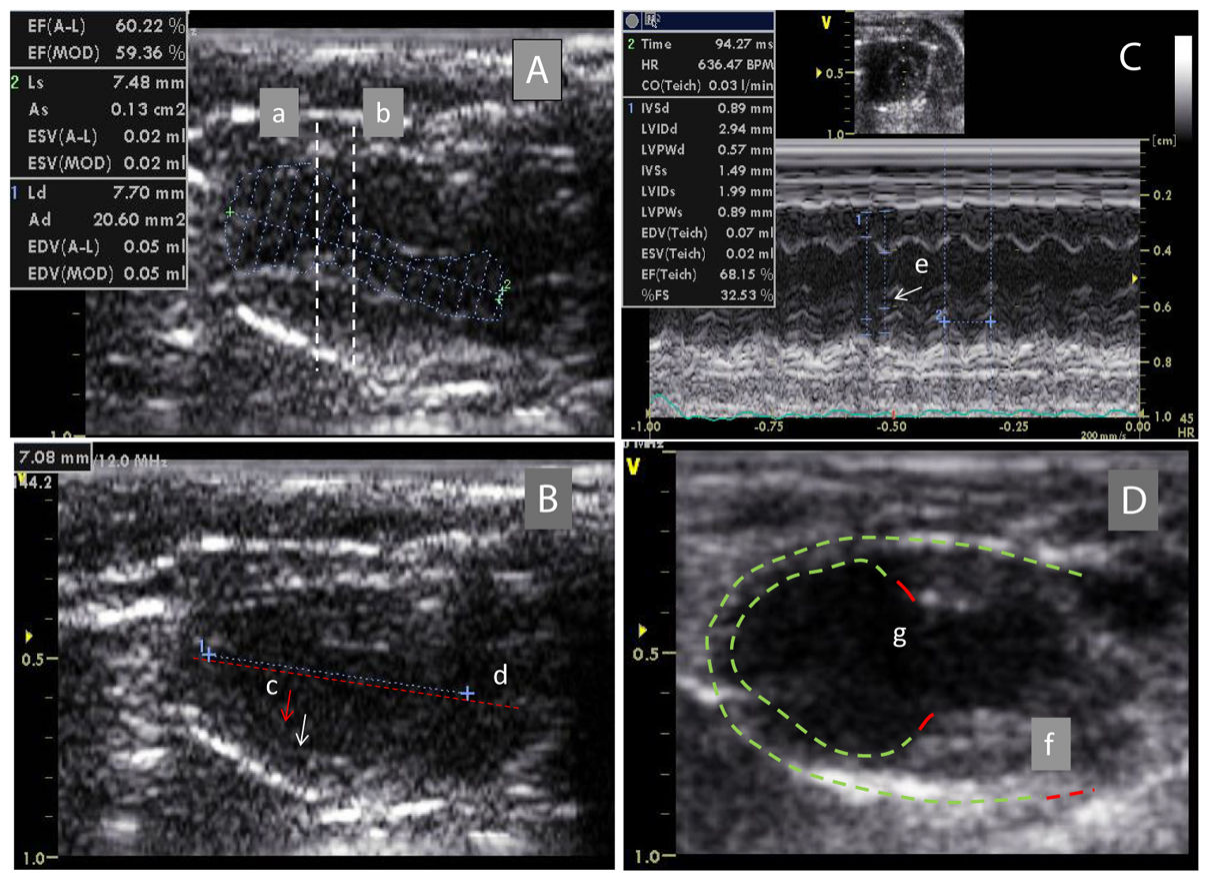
Potential causes of variability of functional and morphological assessment. (A) 2D mode of systolic LV with a MI located at apex. Probe at (a), EF=79%; probe at (b), EF=92%. (B) Diastolic LV echo shows that the internal edge (c; arrows) and end of outflow tract (d; dashed lines) were not clear in some hearts. (C) In M mode echocardiography, the choice of posterior border (g) was difficult in some cases due to hypertrophic papillary muscle. (D) MI area measurement. There is no standard to choose the starting and end point of LV (f) and MI length (g). The boundary between the infarct and normal zone could be identified anywhere along a segment (red line) *in vivo*, leading to measurement variability.
